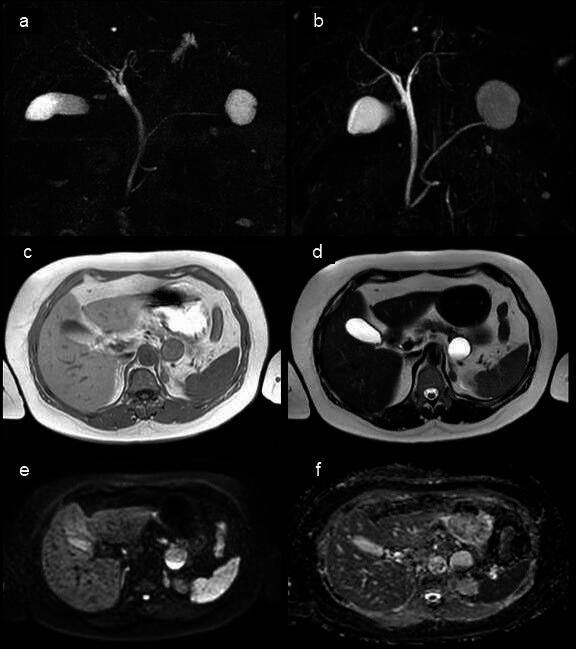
Abdominal magnetic resonance image/cholangiopancreatography (MRI/MRCP). a MRCP at initial examination. b MRCP after 6 months. c T1-weighted image. d T2-weighted image. e Diffusion-weighted image. f ADC-map. A 35 × 30 mm unilocular cyst was observed in the pancreatic tail. The dorsal surface of the cyst was fluid-forming and exhibited high signal on a diffusion-weighted image and low signal on an ADC map
